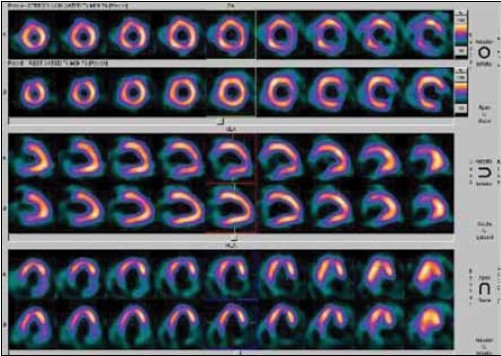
Stress MPI images of a 6-year-old male with a large lateral wall LV aneurysm. Stress SPECT images show moderate reversible perfusion defects in apex and inferior segments
